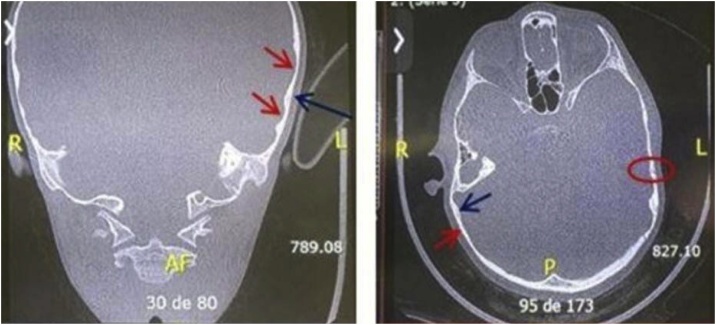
Examples of CT images showing the presence of anatomical factors that could compromise the osseointegration. It is possible to identify bone thickness variability (red arrows), narrow bone thickness (blue arrows) and cranial bone sutures (circle).
